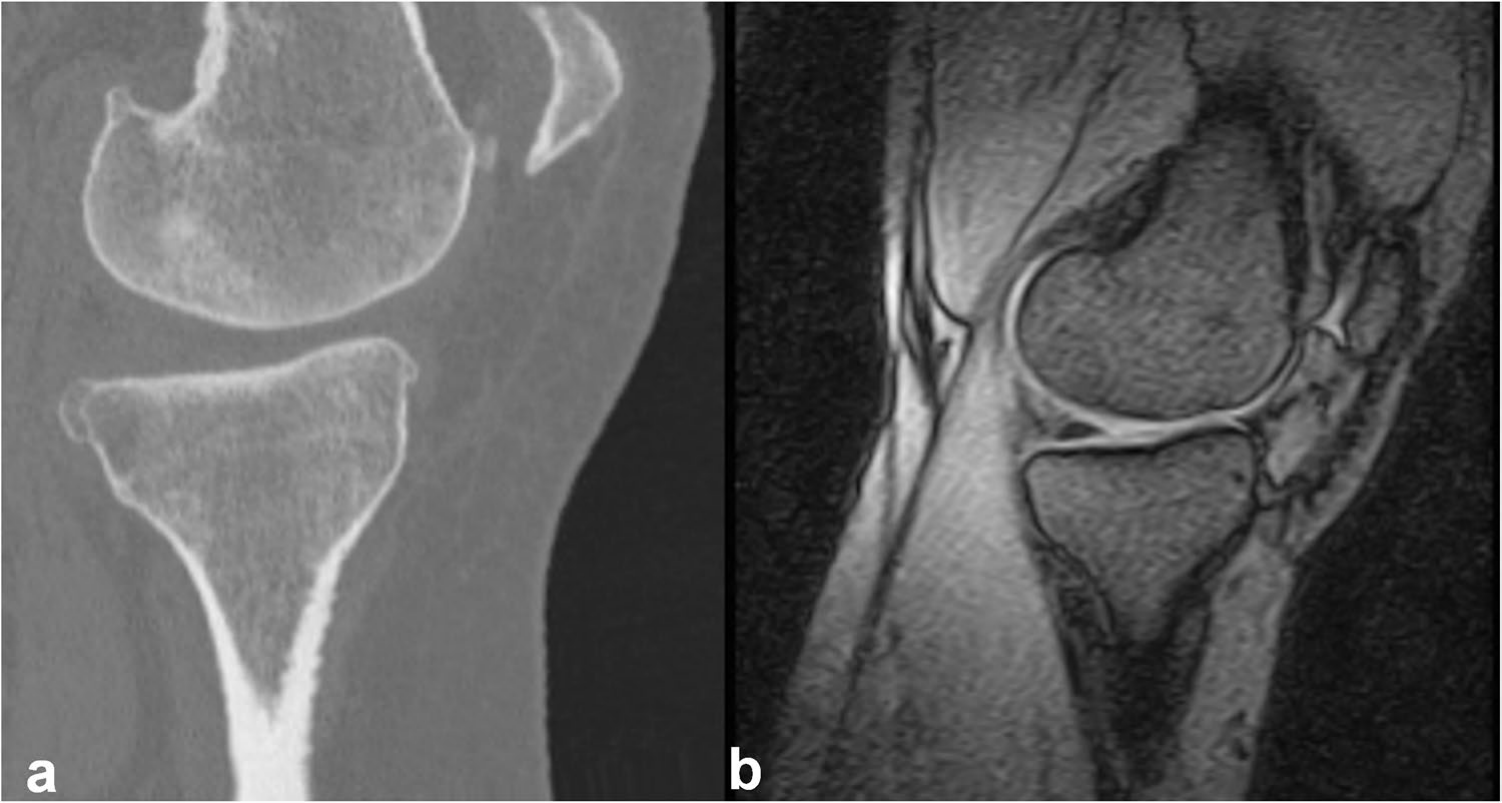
Comparison of re-aligned CT and generic standing MR position. (a) Re-aligned CT image and (b) Standing MR image. CT images were re-aligned such that the new alignments were similar to generic MR standing images.
